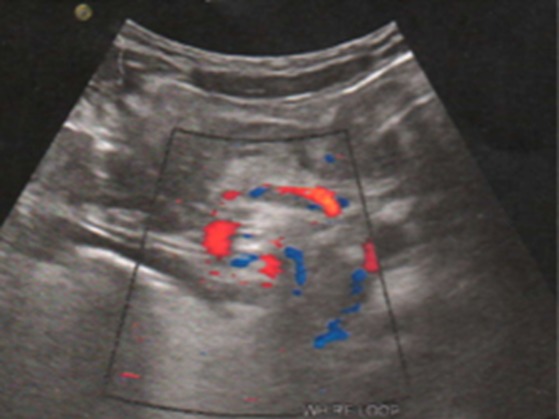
Coupe transversale d’échographie abdominale avec sonde convexe en mode B couplé au doppler montrant une image de tourbillon (« whirl sign ») autour des vaisseaux mésentériques en rapport avec un volvulus
